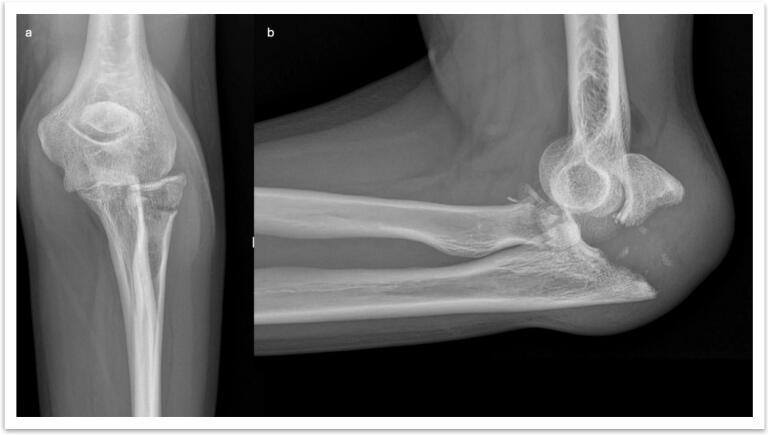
Terrible tetrad of the elbow; a) AP X-ray of the left elbow; b) Lateral X-ray of the right elbow showing the terrible tetrad of the elbow.
